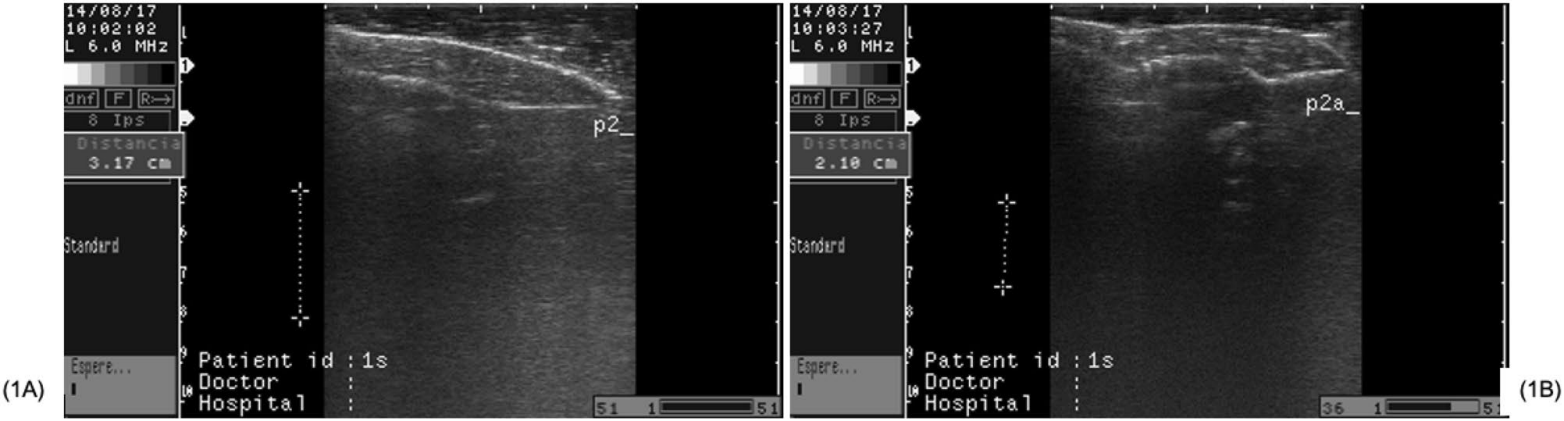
The ultrasound images of M. *pectoralis major*, in domestic quail (*Coturnix japonica*). (A) Longitudinal positions; (B) Transverse positions.
